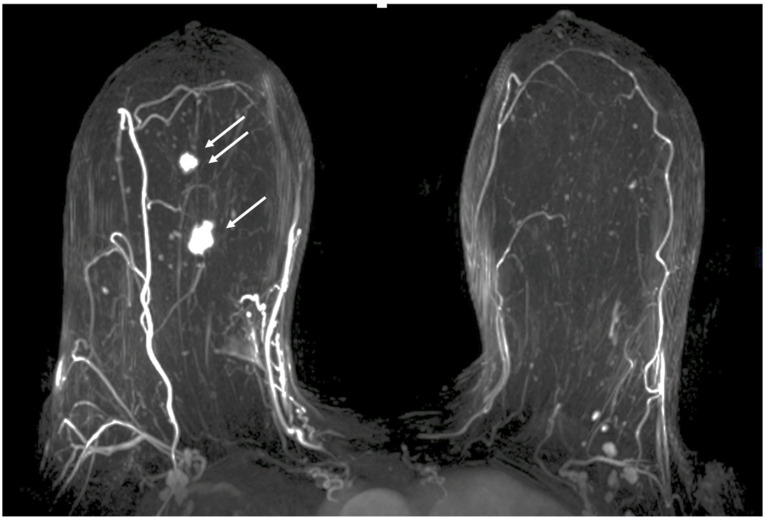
Contrast-enhanced breast MRI image showing multifocal disease indicated by mass-like enhancement of dominant mass (indicated by single arrow and encircled with hash marks) as well as mass-like enhancement of a second focus of disease (indicated by double arrows and encircled with hash marks). The second focus was mammographically-occult.
